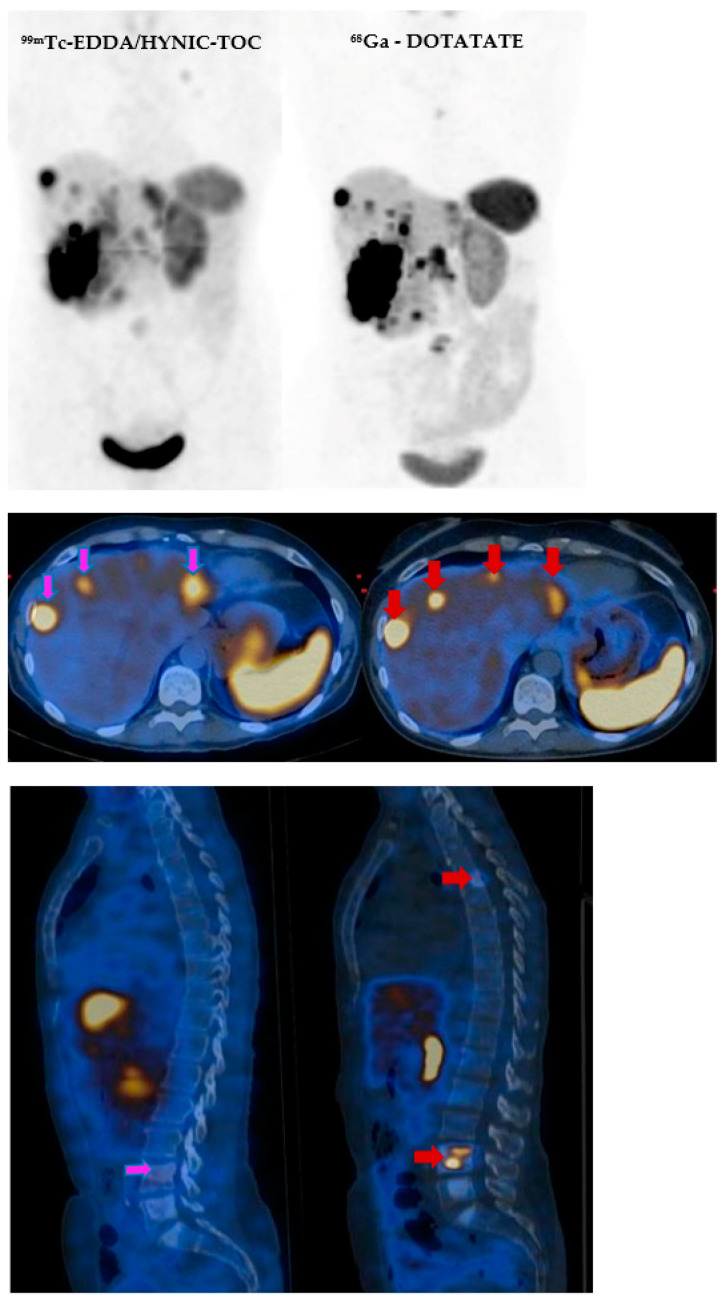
Comparison between ^99m^Tc-EDDA/HYNIC-TOC SPECT/CT (pink arrows) and ^68^Ga-DOTATATE PET/CT (red arrows) in a 54-year-old female patient, diagnosed with a pancreatic neuroendocrine tumor (G2), with multiple liver, lymphatic, and osseous metastases. The patient underwent four sessions of ^177^Lu-DOTATATE PRRT between the PET scan and the SPECT examination. A better resolution for the ^68^Ga-DOTATATE study can be observed, highlighting its higher capacity to detect smaller hepatic lesions and bone metastases. However, similar results can be seen both on ^99m^Tc-EDDA/HYNIC-TOC SPECT/CT and ^68^Ga-DOTATATE PET/CT for bigger lesions.
